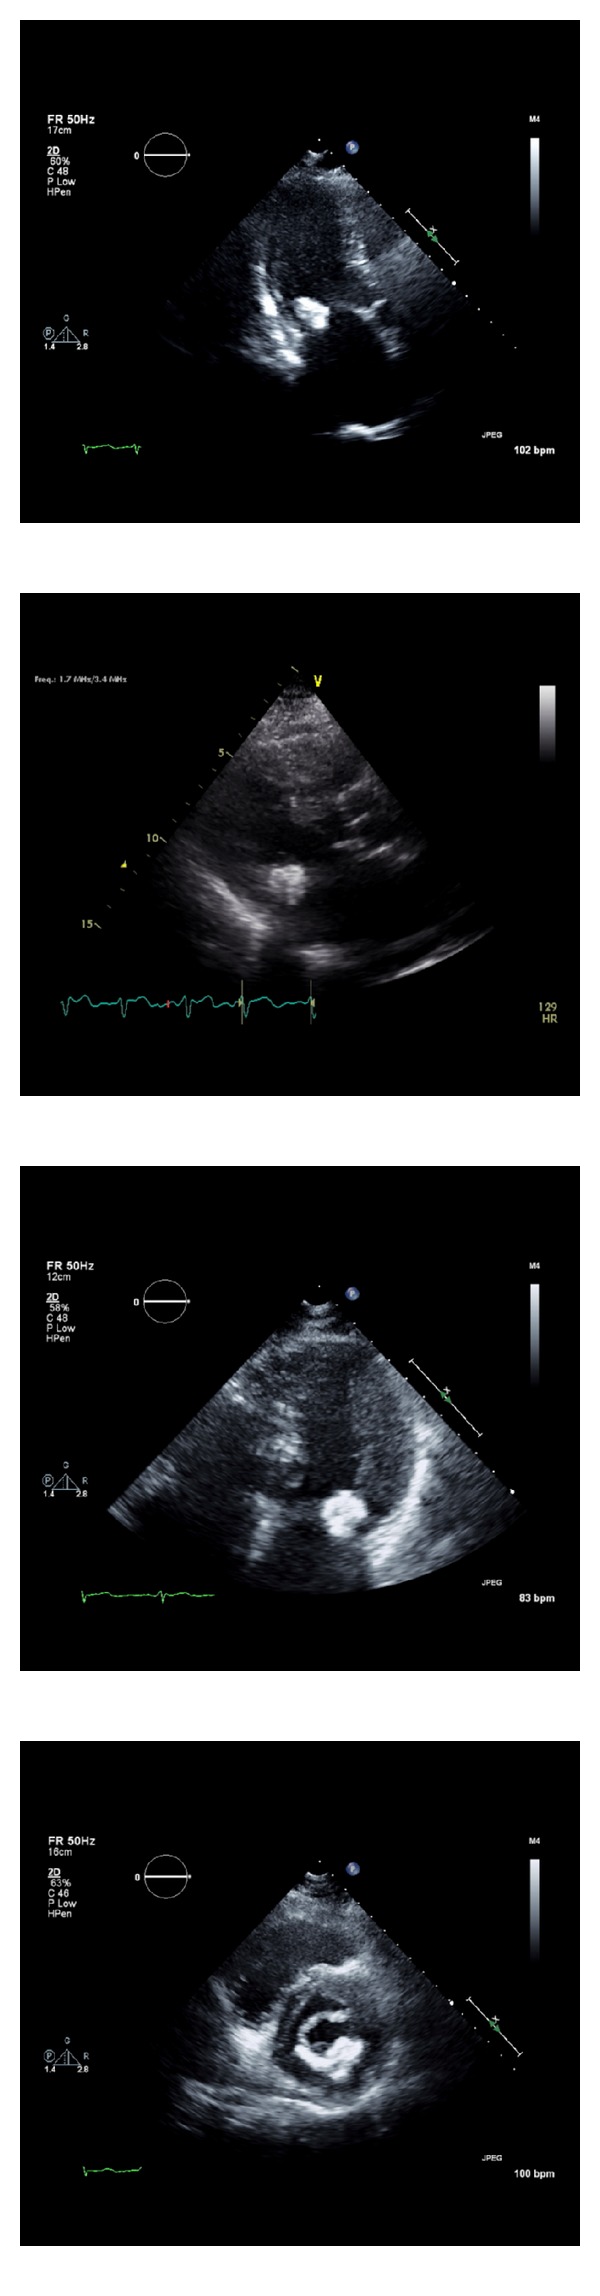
Transthoracic echocardiogram views. Four standard views that show a round echodense mass measuring 20 × 23 mm along the mitral annulus and attached to the posterior mitral leaflet.
